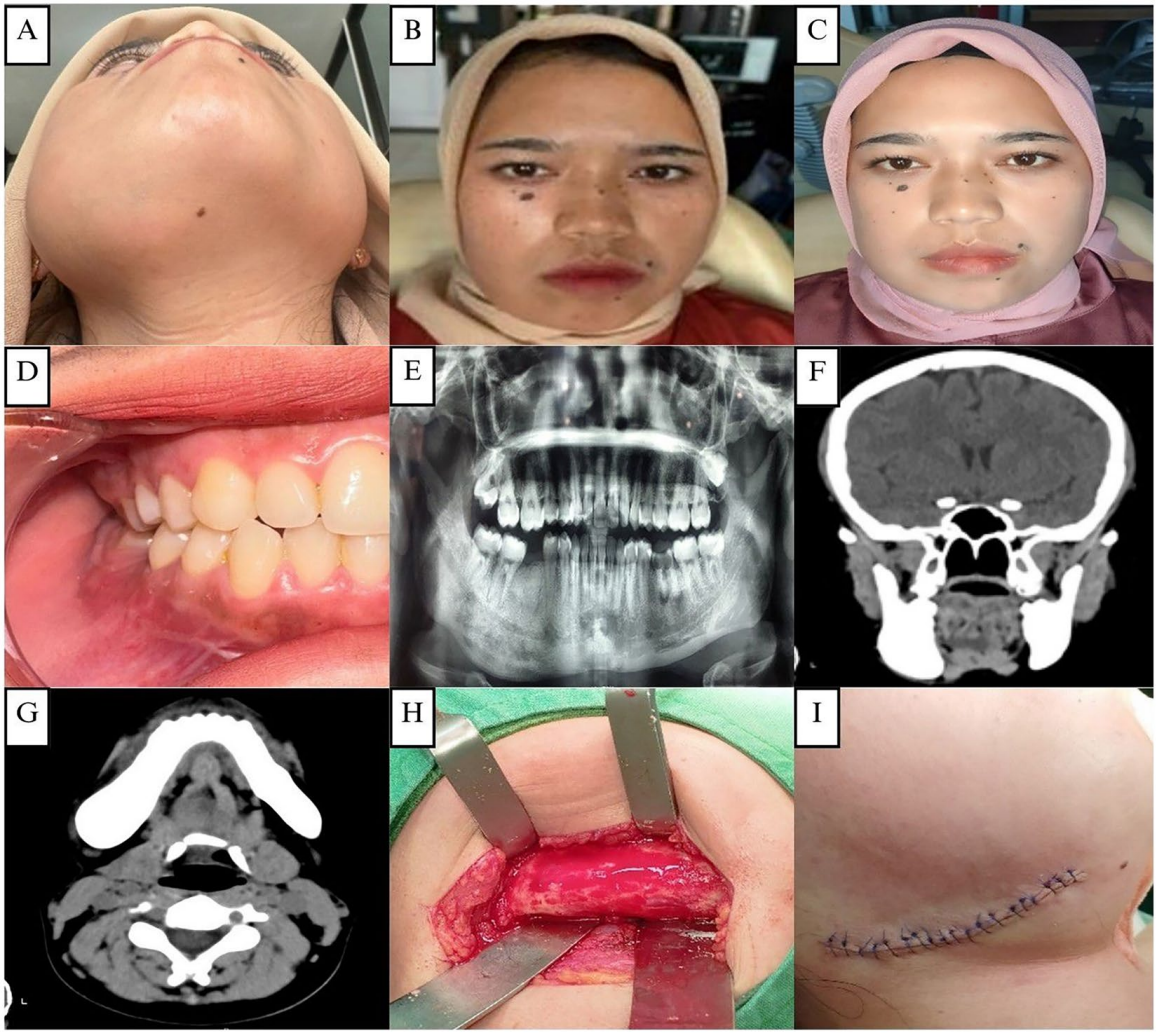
(A and B) The third case, a 23-year-old adult female patient with swelling in the right mandibular area. (C) Two weeks following the patient’s operation. (D) Pre-operative intraoral clinical appearance (E). OPG showing an enlargement in the right ramus mandibular area with the typical ‘ground glass’ like opacification of the bone, which blended with the adjacent normal bone. (F–G) CT scan showing the size of the right mandibular body is larger than the left, no bone erosion, and periosteal reaction is seen. (H–I) Surgical exposure, bone reshaping, and layers of closure of the right mandibular intraoral area.
